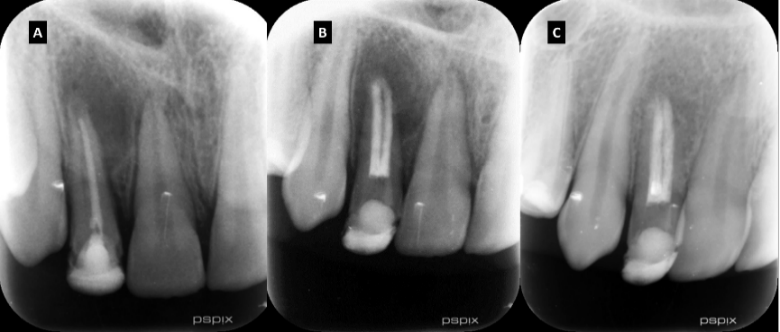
A) Pre-operative radiographic view of tooth #12. Note the large periapical lesion extended coronally on the mesial side of the root, the immature apex of the tooth, and the inadequate root canal treatment; B) After retreatment and coronal restoration. The apical third of both canals obturated with MTA and the rest of the root canal spaces obturated with warm vertical compaction of gutta-percha; C) 18-months follow up tooth #12. Please not the complete osseous healing of the periapical lesion
